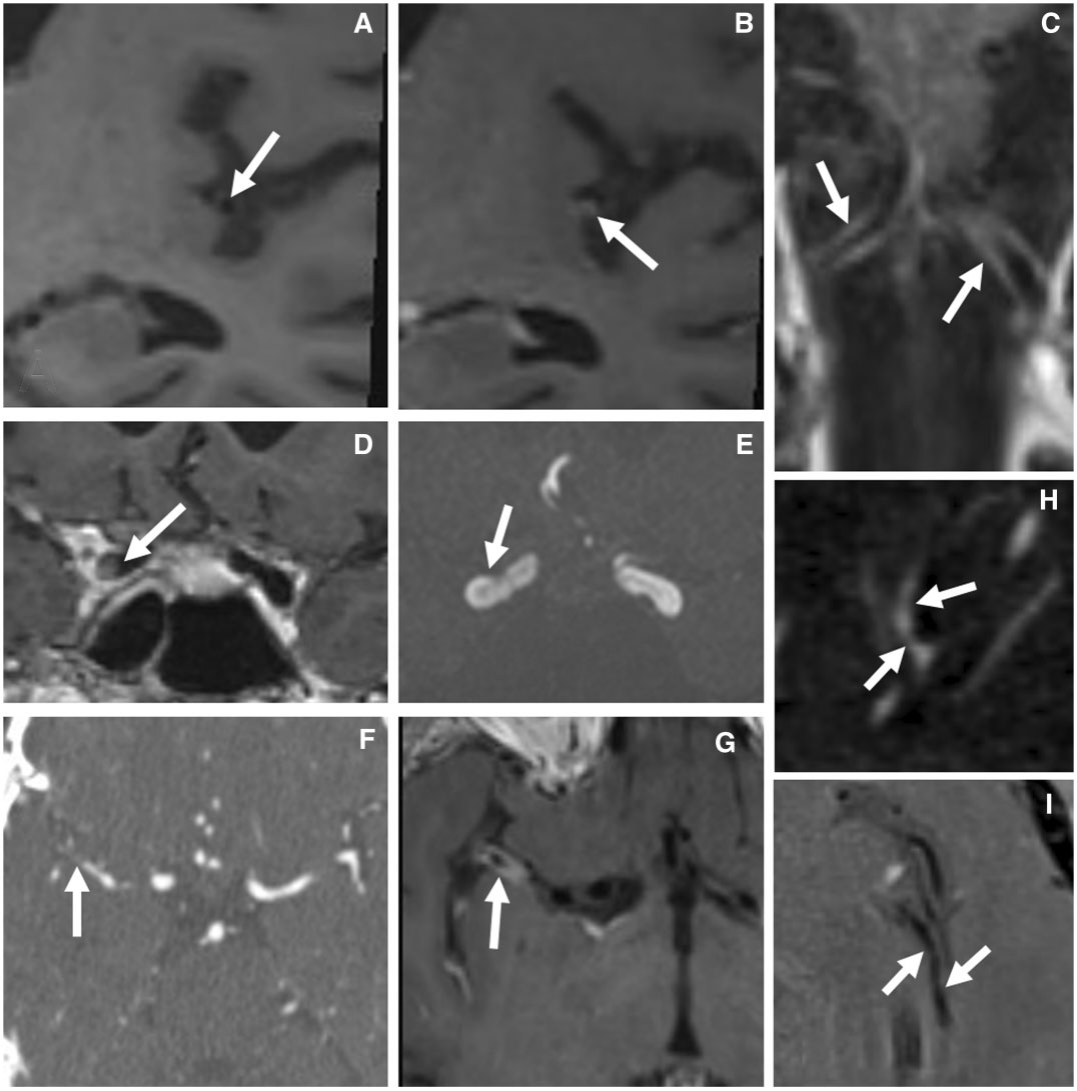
Mimics of CNS vasculitis seen with magnetic resonance vessel wall imaging (MR-VWI). (A, B) Before (A) and after (B) T1 SPACE high-resolution MR-VWI showing an area of eccentric M2 wall thickening and enhancement (arrow) related to non-inflammatory atherosclerotic changes. (C) Post-gadolinium coronal T1 SPACE MR-VWI showing vasa vasorum enhancement of the proximal V4 segments in atherosclerotic disease (often mistaken as inflammation). (D–G) Images representing distal M1 thrombosis with a finding of circumferential enhancement (G) secondary to thrombectemy: area of apparent filling defect in the right cavernous ICA segment (D, arrow) owing to flow-related artefact, with E showing patency on time-of-flight (TOF) magnetic resonance angiography; CTA demonstrating acute distal right M1 occlusion (F, arrow) and G showing MR-VWI following mechanical thrombectomy, with circumferential enhancement thought to be related to mechanical manipulation and disruption of the endothelium. (H, I) Images from a patient with reversible cerebral vasoconstriction syndrome with multifocal areas of luminal narrowing (H) of the proximal M2 on CTA (arrows) and absence of vessel wall enhancement (I) in the same region on MR-VWI suggesting a diagnosis of reversible cerebral vasoconstriction syndrome. CNSV: CNS vasculitis; CTA: CT angiography
